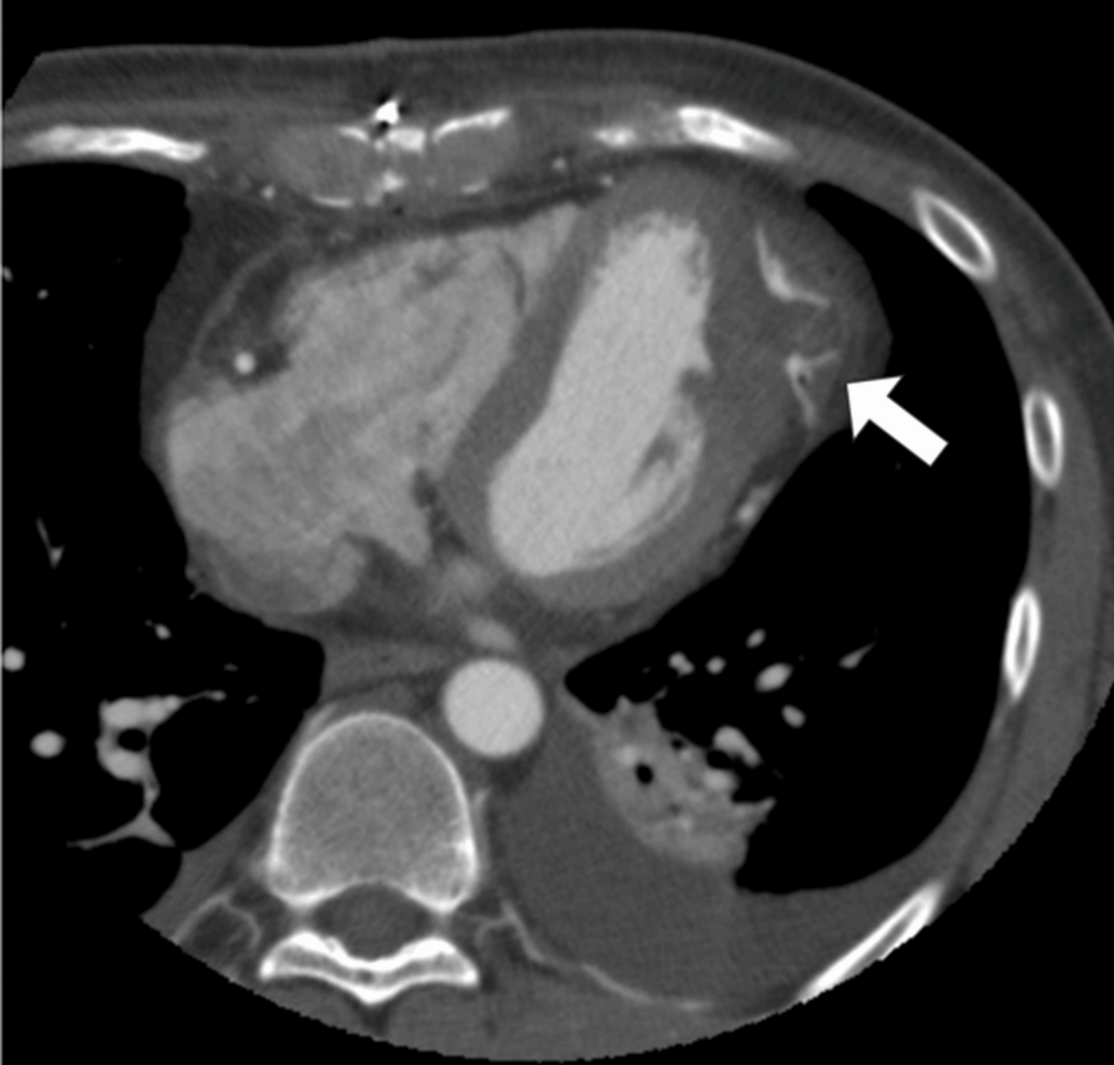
Postoperative contrast-enhanced CT in the axial plane The pseudoaneurysm is completely sealed with no leakage (white arrow). The left ventricular geometry is preserved.
